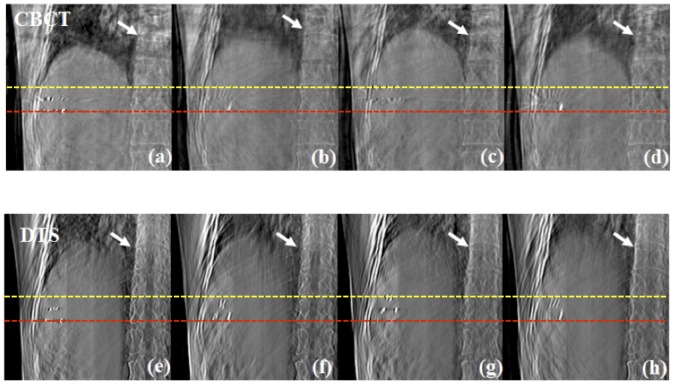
Comparative result (Coronal view) between 4D CBCT and DTS of liver cancer patient #1 case with peak-exhale (a), (e), mid-inhale (b), (f), peak-inhale (c), (g) and mid-exhale (d), (h) phases.
